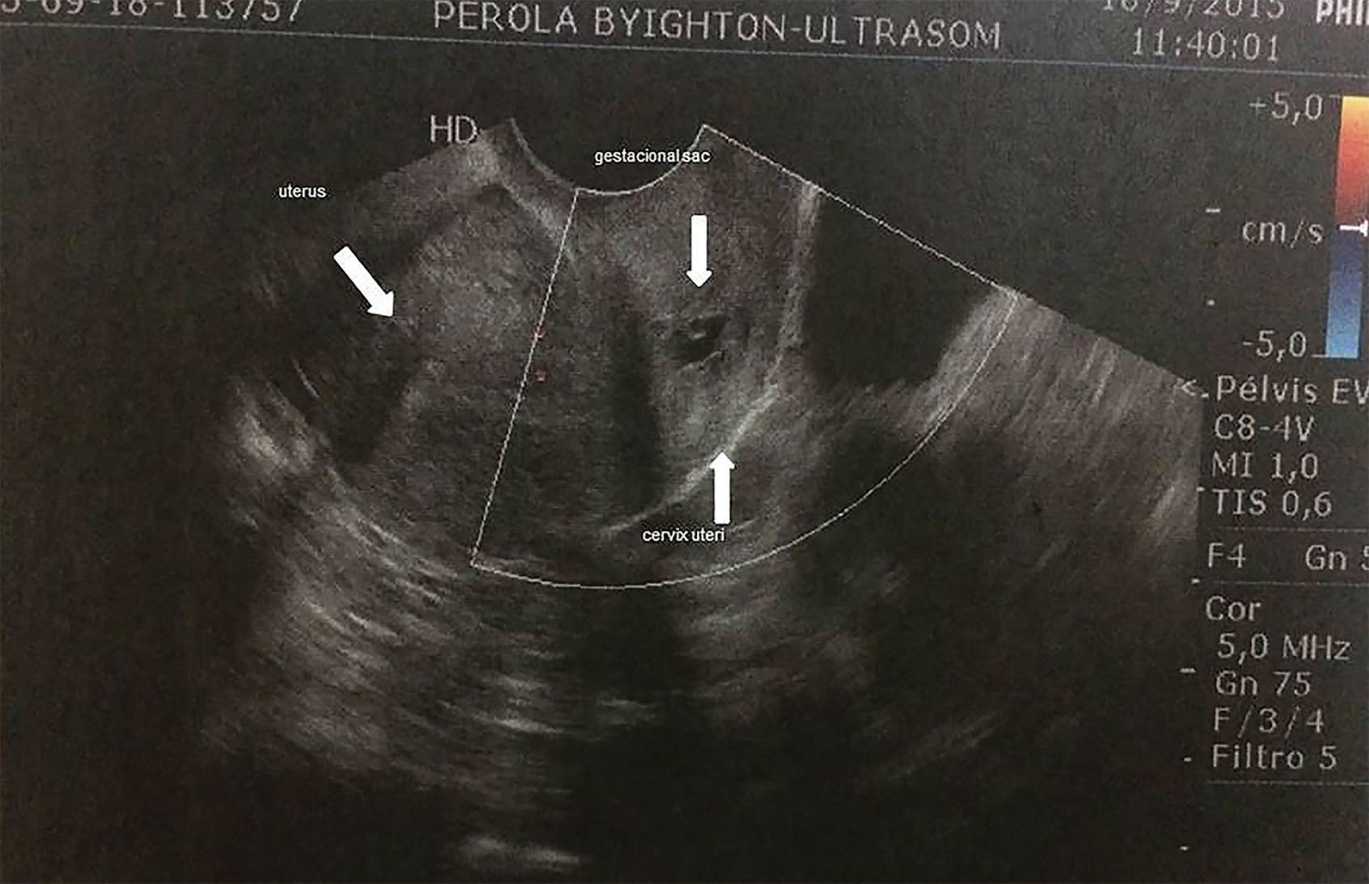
Transvaginal ultrasonography identifying the ectopic pregnancy presenting
